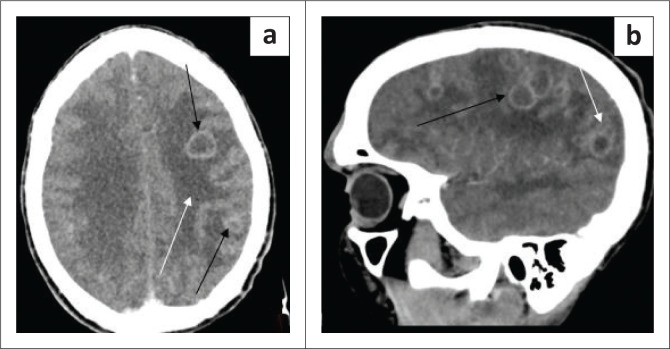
(a) Axial post-contrast computed tomography (CT) brain demonstrates vasogenic oedema (white arrow) and rim-enhancing lesions in the left parietal lobe (black arrows). (b) Sagittal post-contrast brain CT demonstrated loculated rim-enhancing lesions (black arrow), of which some are bilobed (white arrow).
